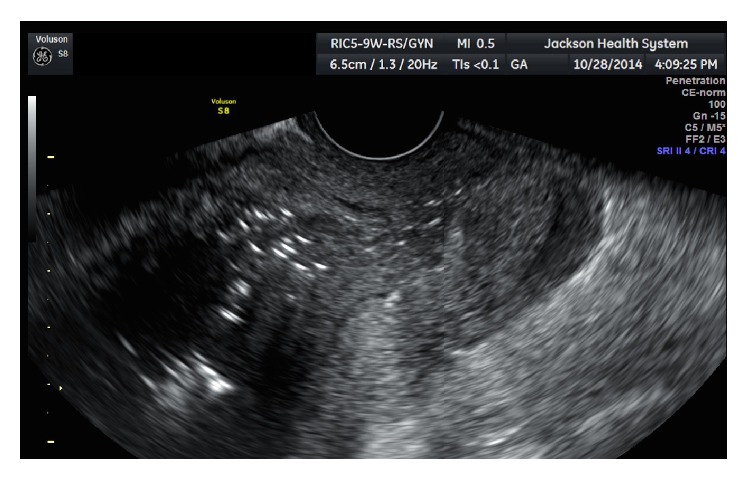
Ultrasound showing gas inside the endometrial cavity.
